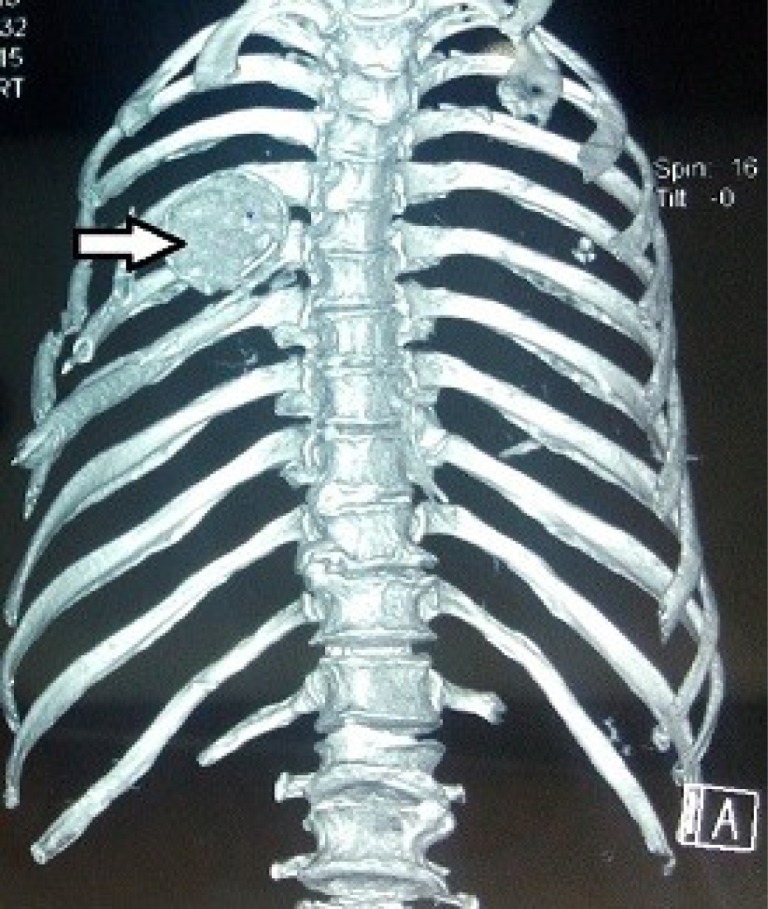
3D reconstruction CT showing right sided intrathoracic humeral head with fractured ribs on ipsilateral side.
